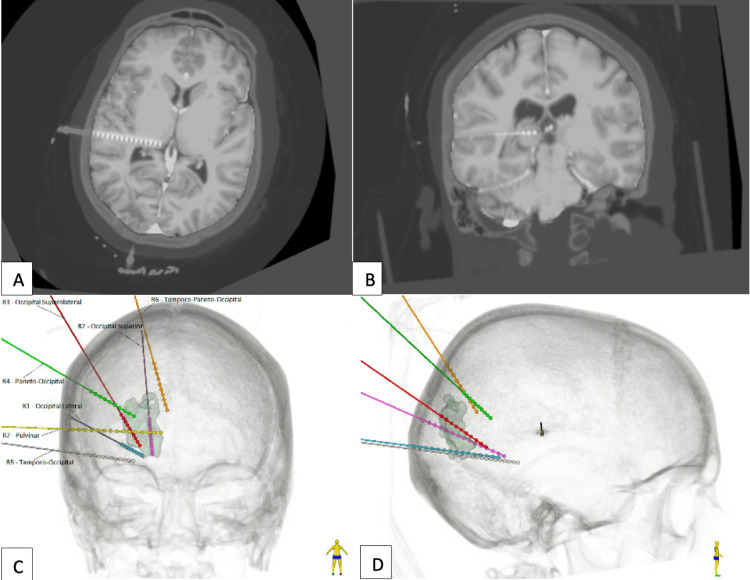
Axial (A) and coronal (B) views of MRI brain with a superimposed SEEG depth electrode implanted in the right pulvinar nucleus. Coronal (C) and (D) sagittal three-dimensional reconstruction of CT brain demonstrating implanted SEEG depth electrodes. SEEG: stereoelectroencephalography
